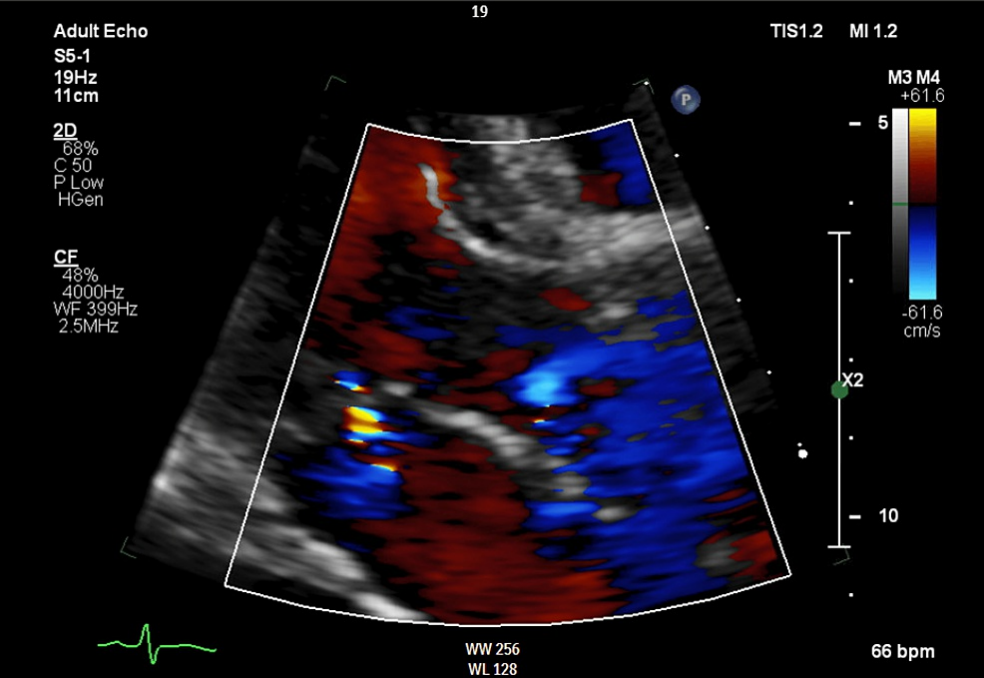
Transesophageal echocardiogram showing showing severe mitral regurgitation at the end of systole
